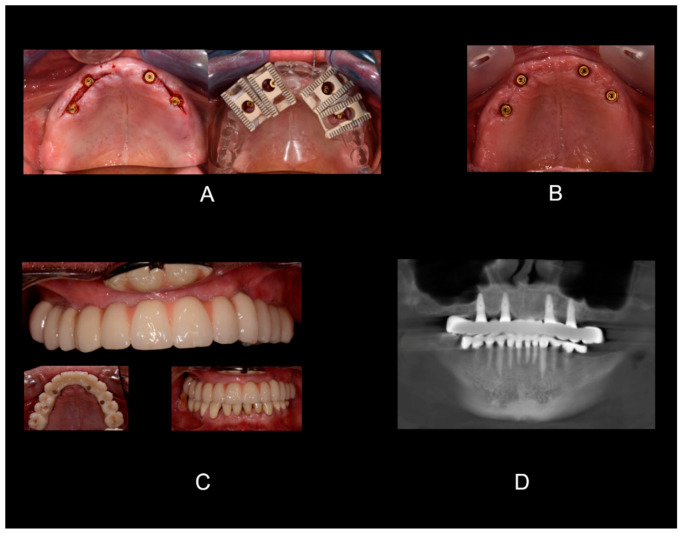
Prosthetic rehabilitation and postoperative follow-up. Sequential steps in the maxillary prosthetic phase following implant integration. (A) Placement of compact conical abutments on maxillary implants after a 6-month healing period, ensuring uniform emergence profile for prosthetic connection; (B) three-week postoperative control showing satisfactory soft tissue healing and abutment stability; (C) delivery of the definitive zirconia bridge on four maxillary implants with passive fit and functional occlusion; (D) one-year postoperative radiographic evaluation showing stable osseointegration and successful prosthetic integration.
